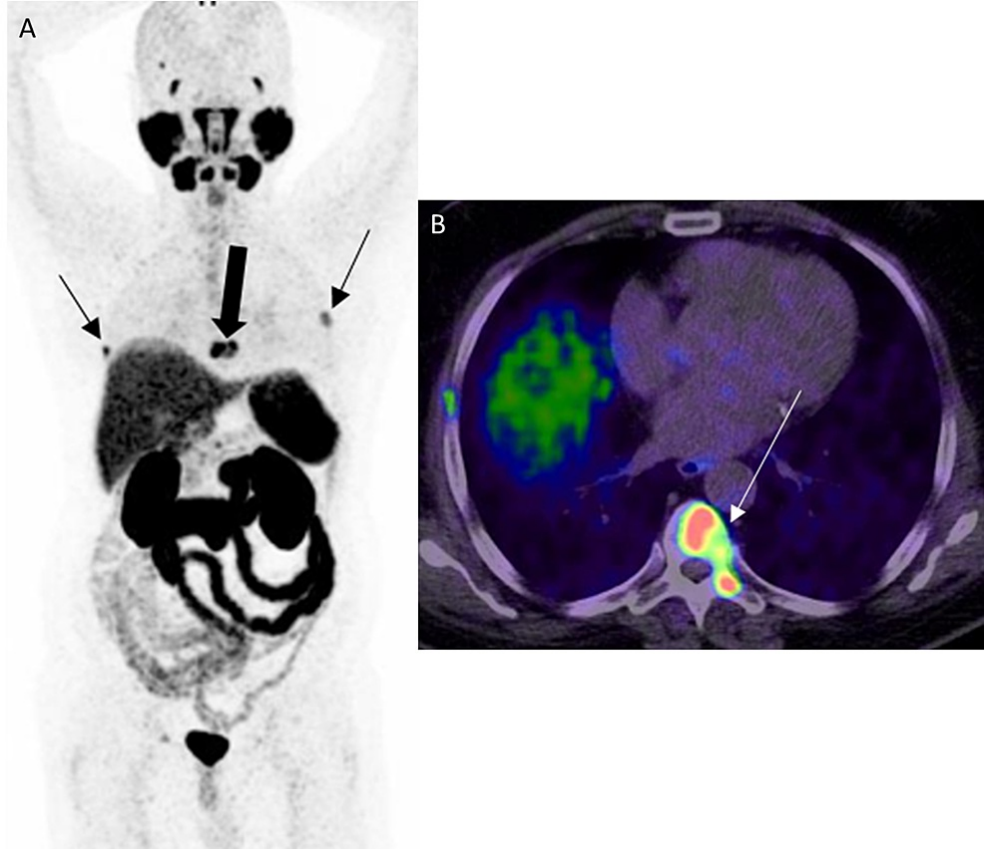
Bone metastases A: 68Ga PSMA PET MIP image shows metastatic bone lesions with focal uptake in bilateral ribs (black thin arrows) and a thoracic vertebra (black thick arrow). B: PET/CT axial image shows intense uptake in the metastatic thoracic vertebra lesion (white arrow) in the same patient.
